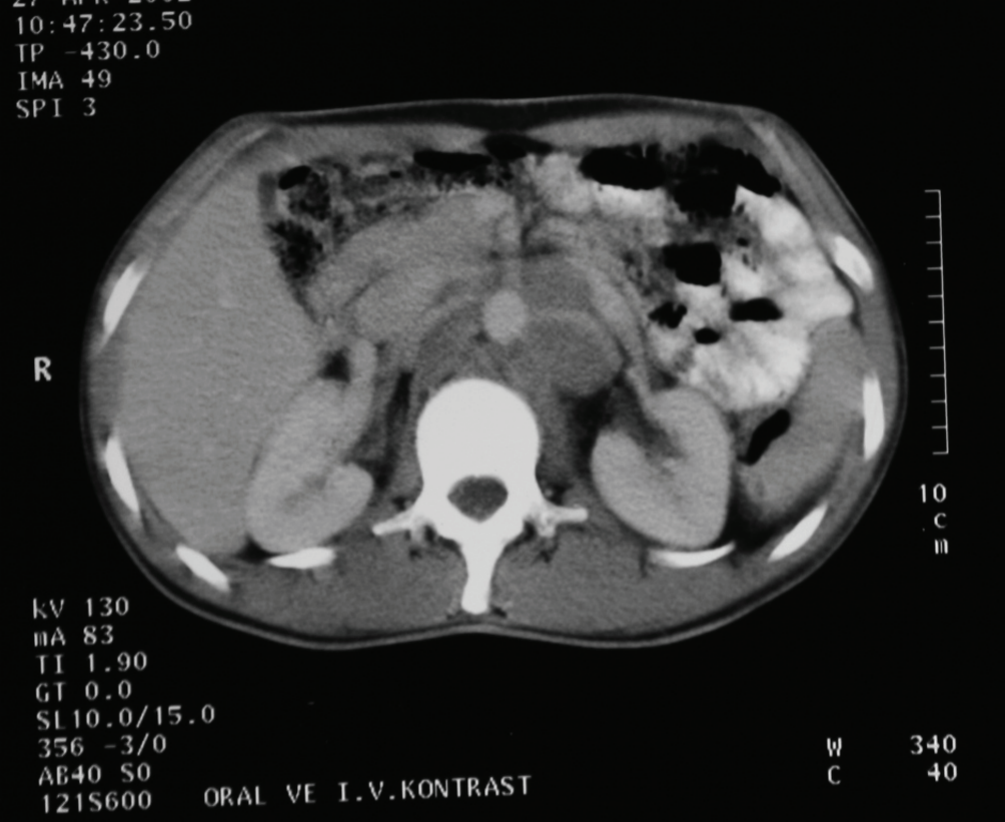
After the four courses of chemotherapy treatment, computed tomography showed that the abdominal mass had regressed.
